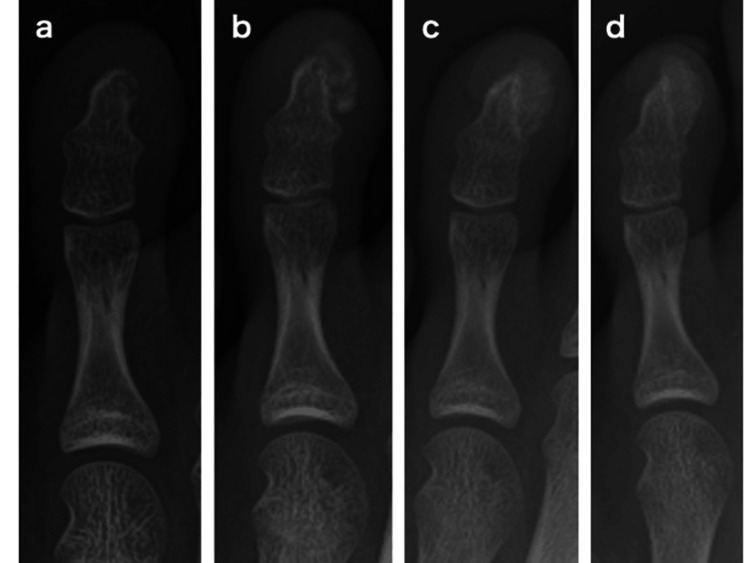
Postoperative X-rays. Postoperative X-rays, at 1 week (a), 4 weeks (b), 8 weeks (c), and 12 weeks (d). At around 4 weeks after surgery, radiographs show pale calcification in an area larger than the original bone tumor (b). Thereafter, the calcification gradually expands in the resected area, and at 3 months after surgery, the bone tumor has grown to several times the size of the original tumor (d).
